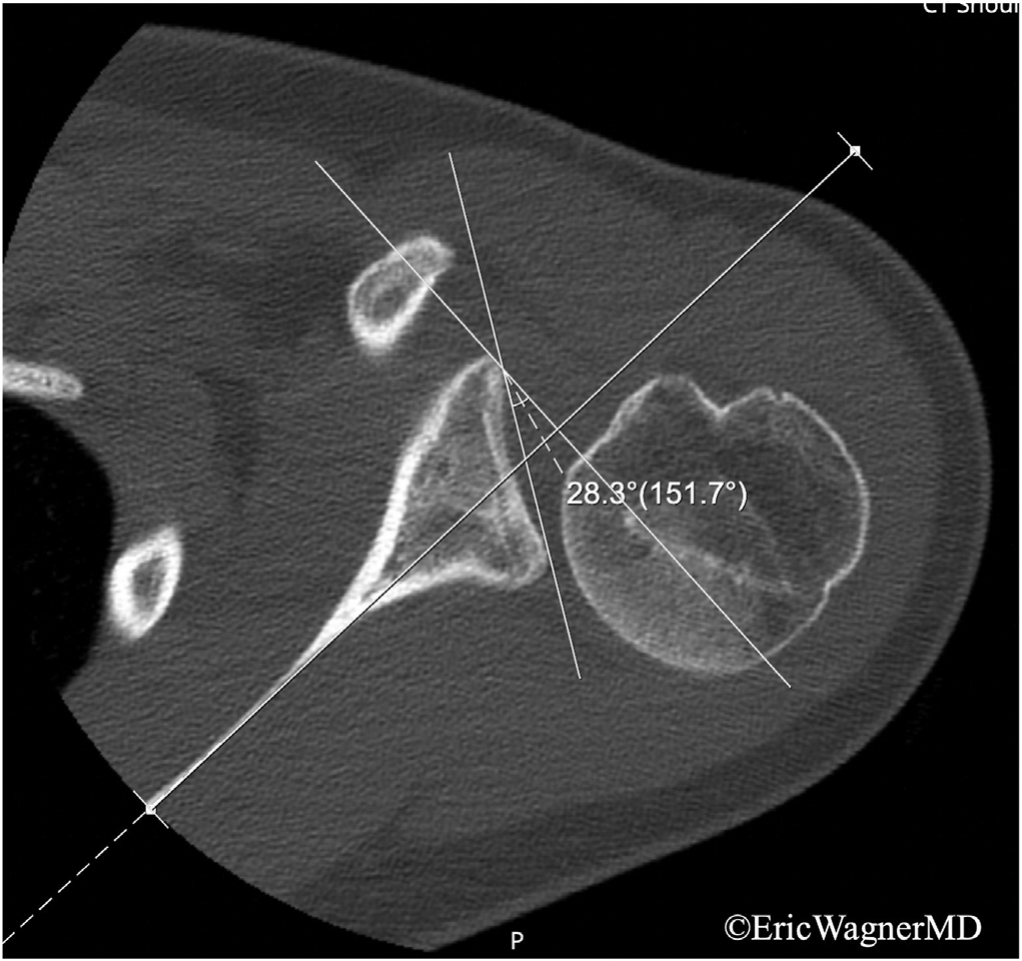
Axial CT image of left glenohumeral joint displaying Walch type B3 dysplastic glenoid with 28.3° of retroversion, and significant posterior humeral head subluxation in the setting of capsulolabral hyperlaxity. *CT*, computed tomography.
